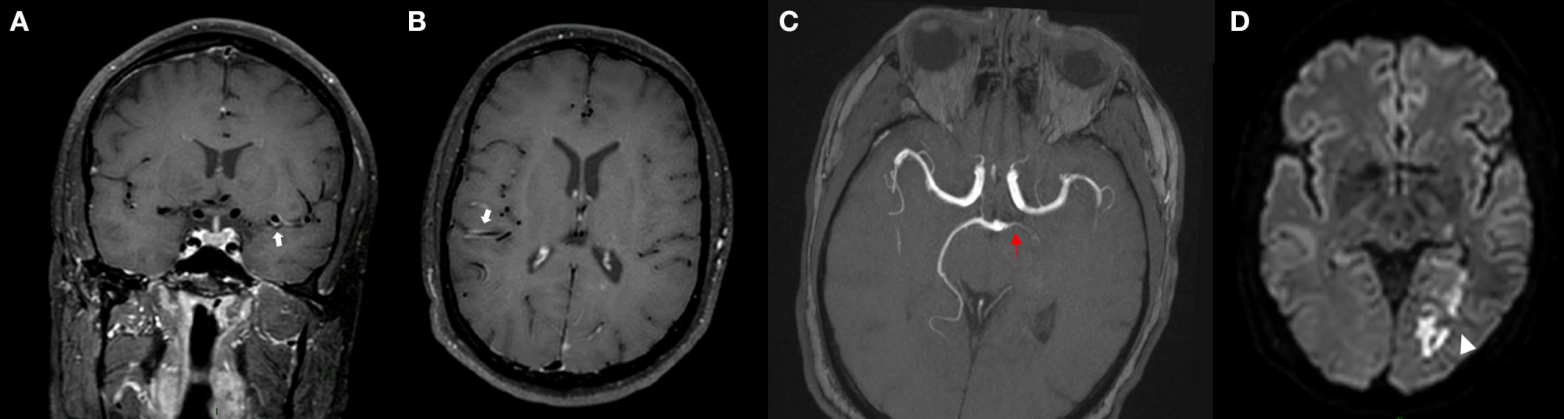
MRI of a patient presenting with PACNS. IVW-MRI showing multiples areas of concentric contrast enhancement in the proximal left and distal right the middle cerebral arteries [(A,B) arrows]. Time of flight-MRA showing a stenotic lesion of the left posterior cerebral artery associated with an acute infarct in the left occipital lobe [(C) red arrow, & (D) arrowhead].
